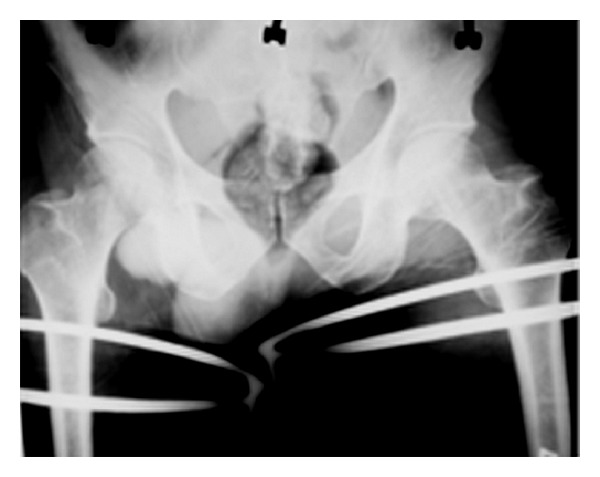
Radiograph of the pelvis of the same patient showing reduction of the dislocated hips.
